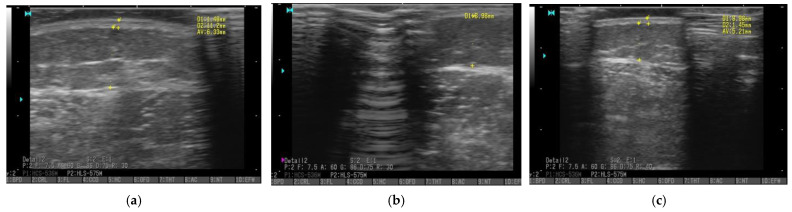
Thickness of adipose tissue measured by ultrasonography at ½ length on the side of the shin on the right leg in Patient 2B at baseline (a), after 4 weeks (b), after 6 weeks (c). The thickness of adipose tissue is marked with † signs, ^1^ and ^2^ measurement stand for the thickness of the skin.
